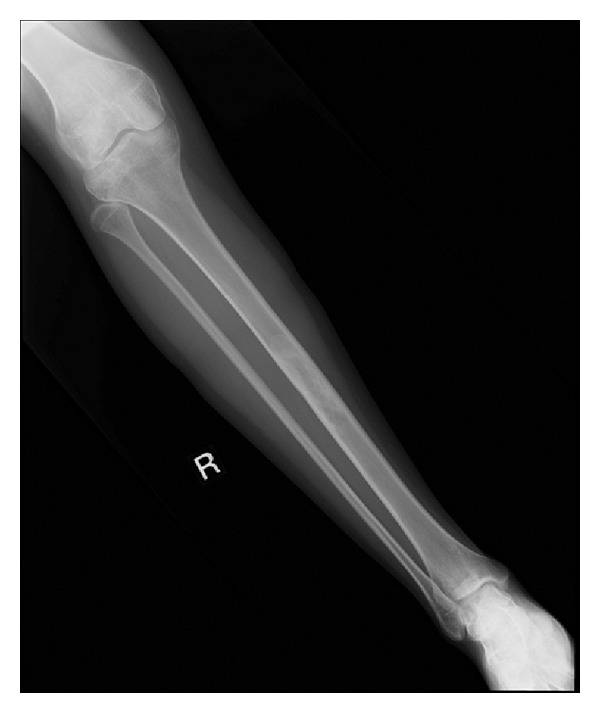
Plain radiographs of the right knee five years after the first surgery.
